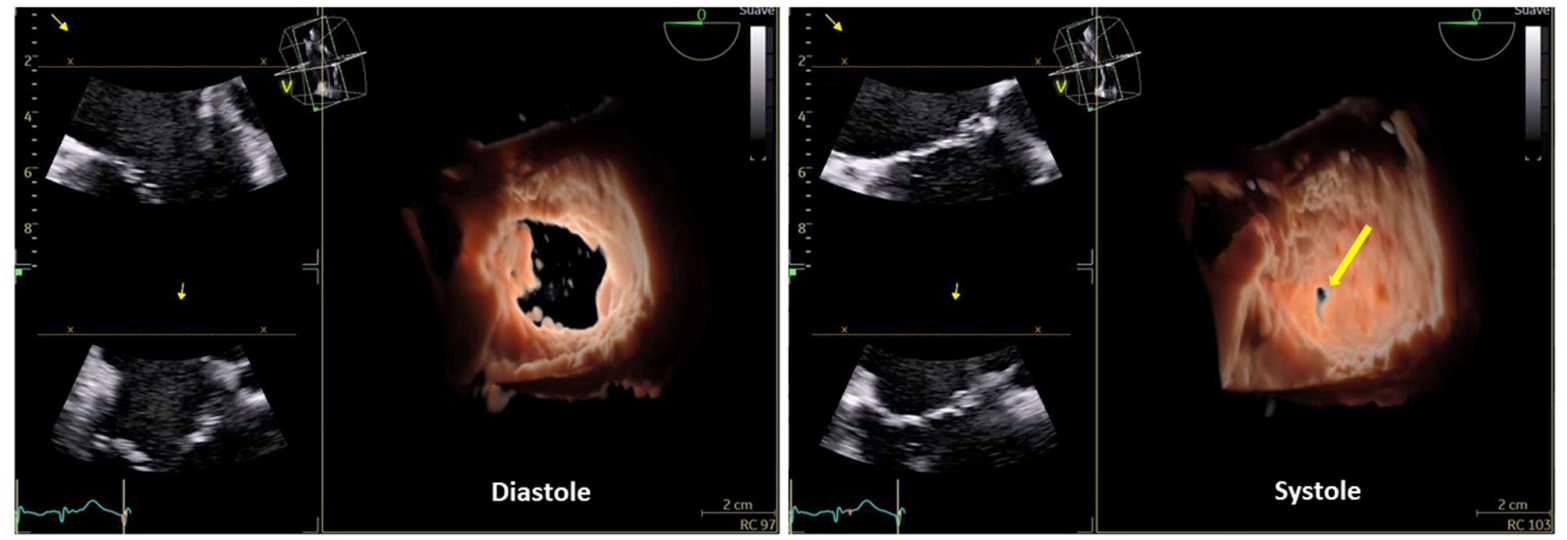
Three-dimensional (3D) echocardiography of the tricuspid valve with front view, from the right atrium in diastole and systole where the central coaptation defect (yellow arrow), due to annular dilation, is seen.
